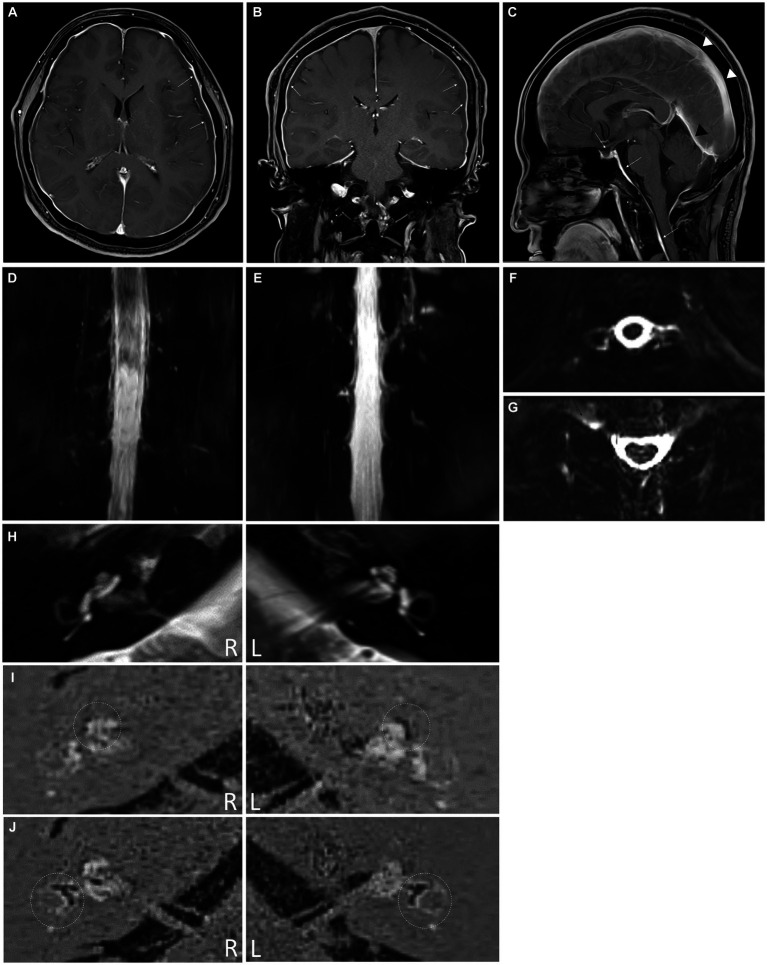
(A) Axial post-contrast medium T_1_-weighted magnetic resonance (MR) image showing diffuse smooth thickening of the cerebral convexity dura (white arrows) and bilateral subdural liquid collection (yellow arrows). (B) Coronal T_1_-weighted MR image with contrast demonstrating diffuse dural enhancement (white arrows) with bilateral subdural liquid collection (yellow arrows). (C) Sagittal post-contrast medium T_1_-weighted MR image showing dural enhancement of the pituitary (yellow arrow). The presence of diffuse smooth dural thickening (white arrows), which extends into the spine, indicates spontaneous intracranial hypotension, demonstrating prominent sagittal sinus (arrowheads) and transverse sinus (black arrowhead). (D–G) Intrathecal Gd-magnetic resonance myelography images revealing cerebrospinal fluid leakage at multiple levels (C4-5, C5-6, C6-7, and C7-T1 on right side, C4-5 on left side, and T1-2 on both sides) in the coronal (D) and axial views (E). (F,G) Cerebrospinal fluid leakage is also observed at the T10-11 and T11-12 levels on both sides. (H) Axial T_2_-weighted magnetic resonance (MR) image showing a normal internal auditory canal in both ears. The HYDROPS MR image was generated by subtracting the positive perilymph signal from the positive perilymph image. The dotted circles indicate the cochlea (I) and vestibular apparatus (J). The endolymphatic hydrops is detectable as a black area inside the perilymphatic space filled with the gadodiamide.
